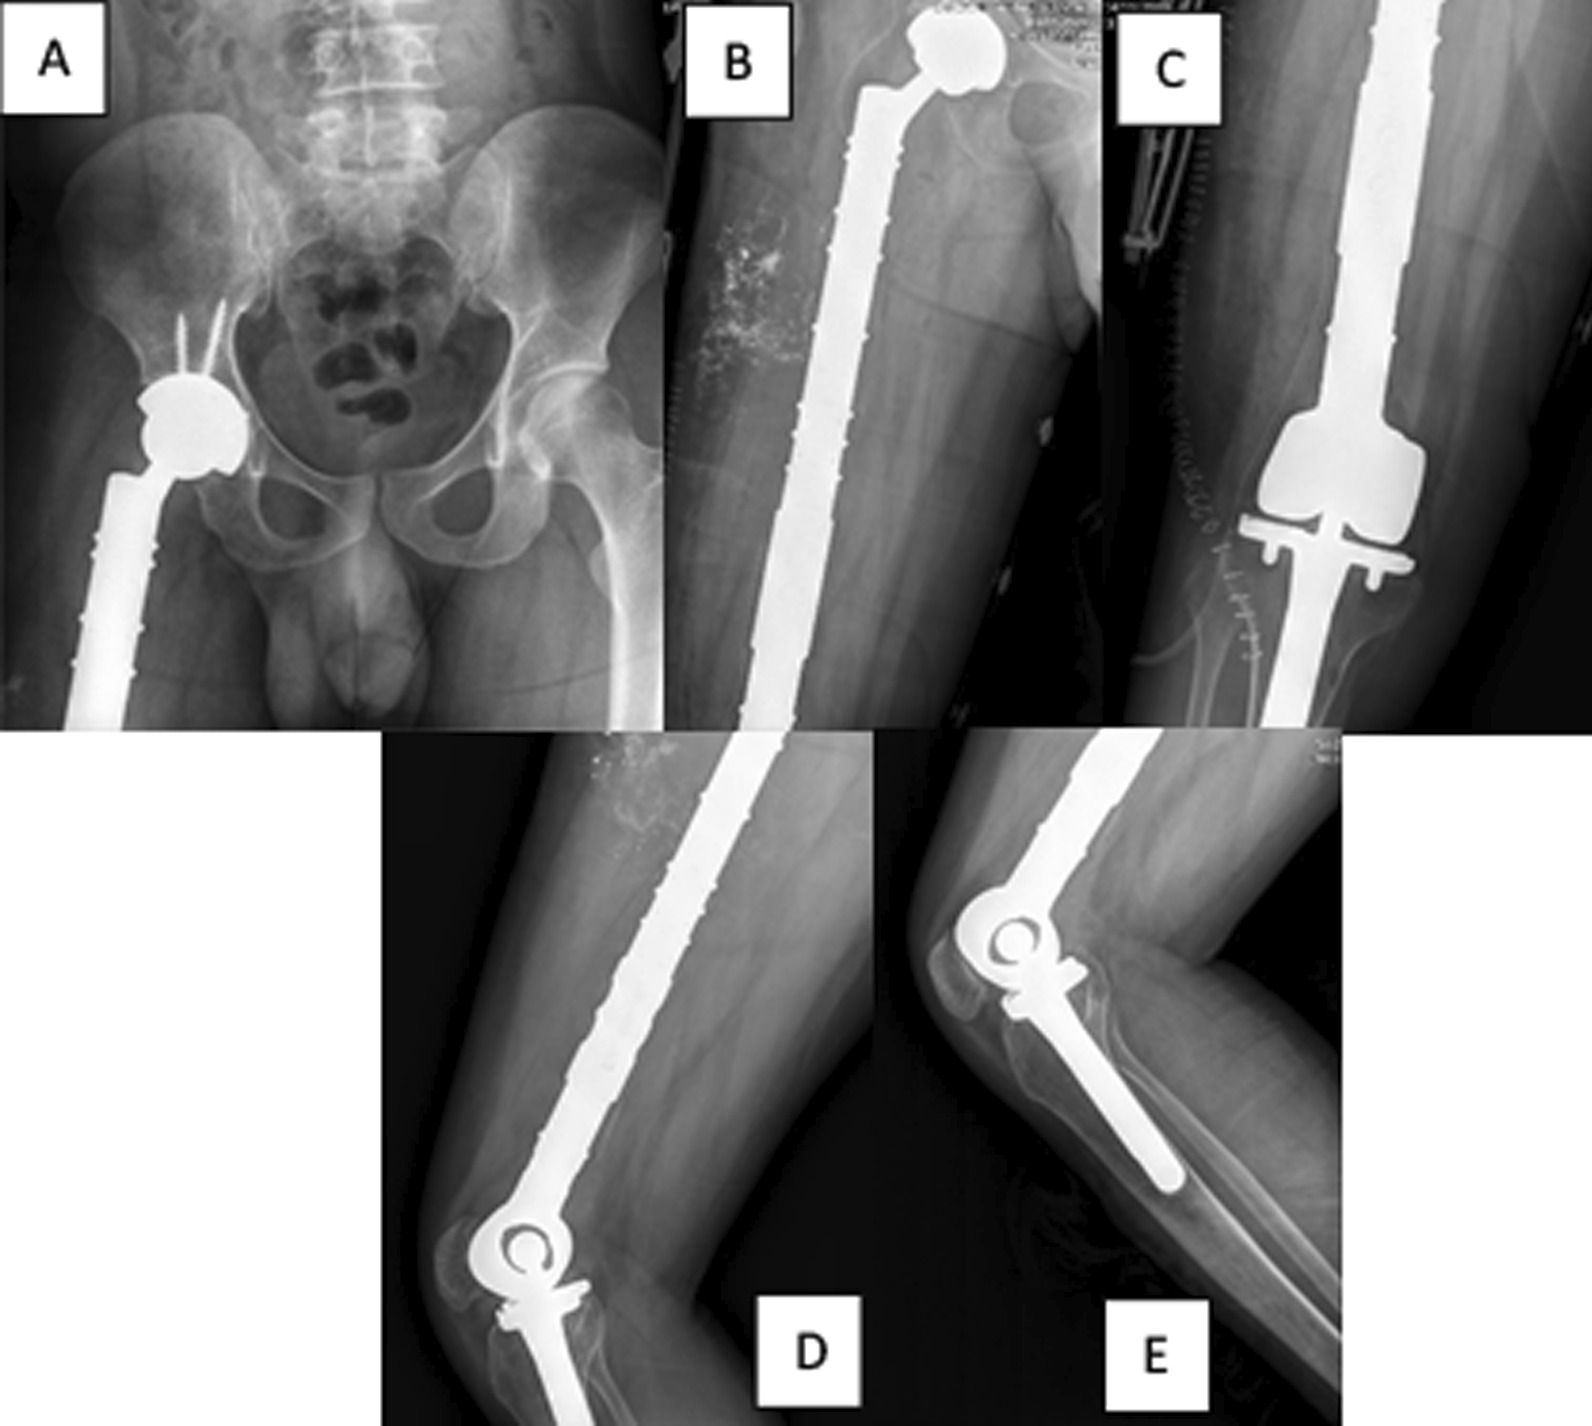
Postoperative radiograph including Anteroposterior view of pelvic (A), femur (B), knee (C), and lateral view of the femur (D) and knee (E)
